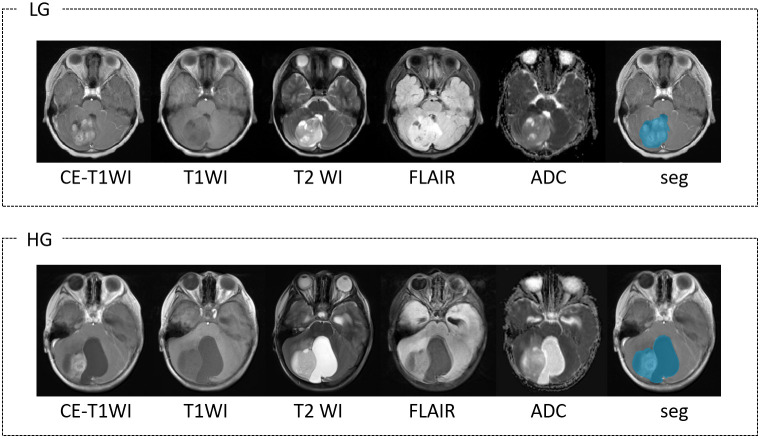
Multi-sequence MRI image comparison of low-grade (LG) and high-grade (HG) brain tumor. Show the CE-T1WI, T1WI, T2WI, FLAIR, ADC sequences and tumor segmentation (Seg) results in sequence, and the blue area represents the tumor area.
